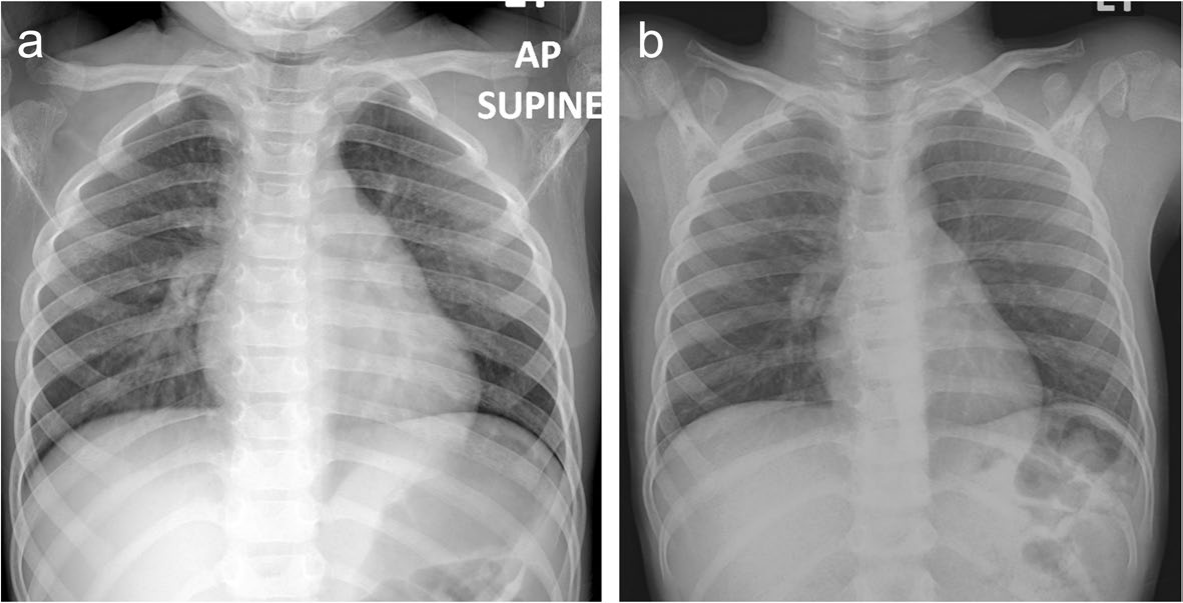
A 4-year-old girl with COVID-19 pneumonia who presented with a low-grade fever. a The initial chest radiograph shows bilateral peribronchial thickening, predominantly in the right lower lung field. The findings are consistent with the indeterminate pattern of COVID-19 pneumonia. The severity scoring in this patient was calculated as the sum of the right lung scores and the left lung scores (total scores = 2 + 1 = 3). b On day 6 of admission, the radiographic evaluation reveals an almost complete resolution of the infiltrative lung involvement. The follow-up severity score in this patient was calculated as the sum of the right lung scores and the left lung scores (total scores = 1 + 0 = 1). COVID-19, coronavirus disease-19
